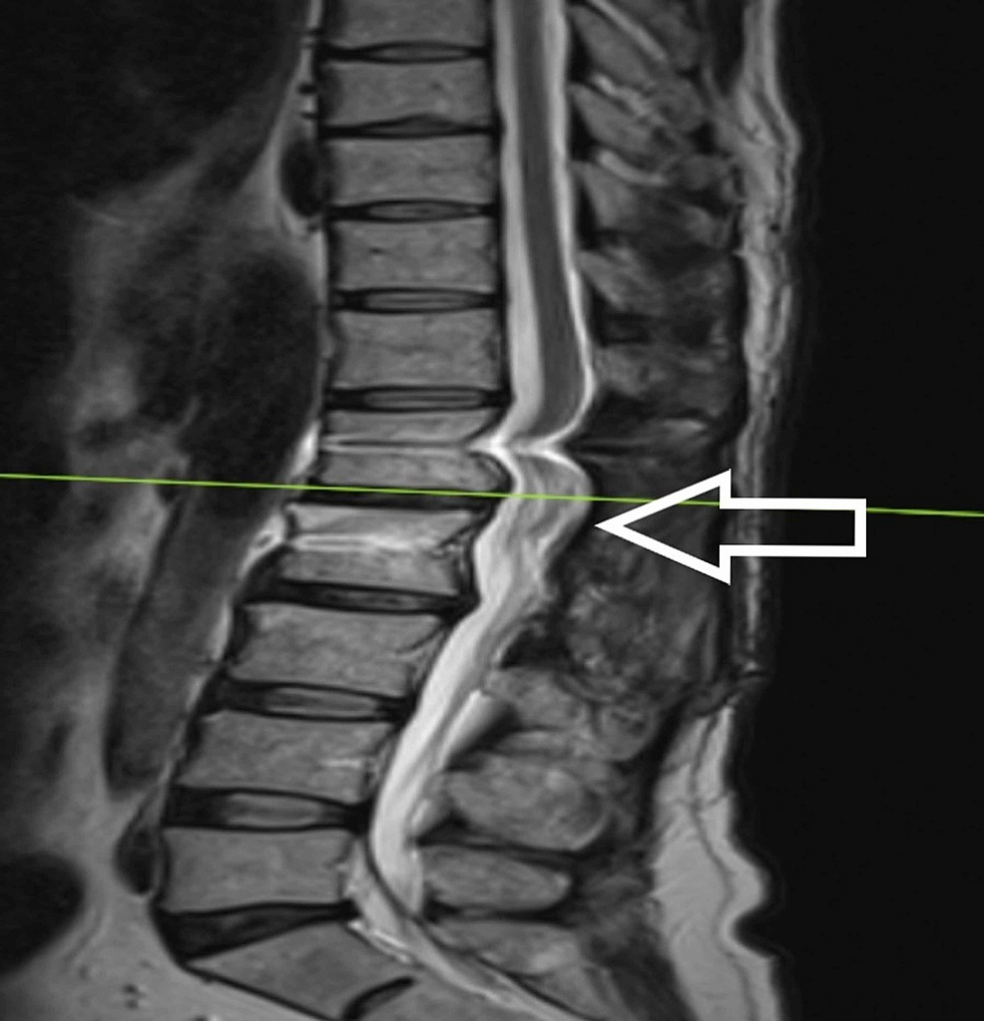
Sagittal MRI after 2.5 years
